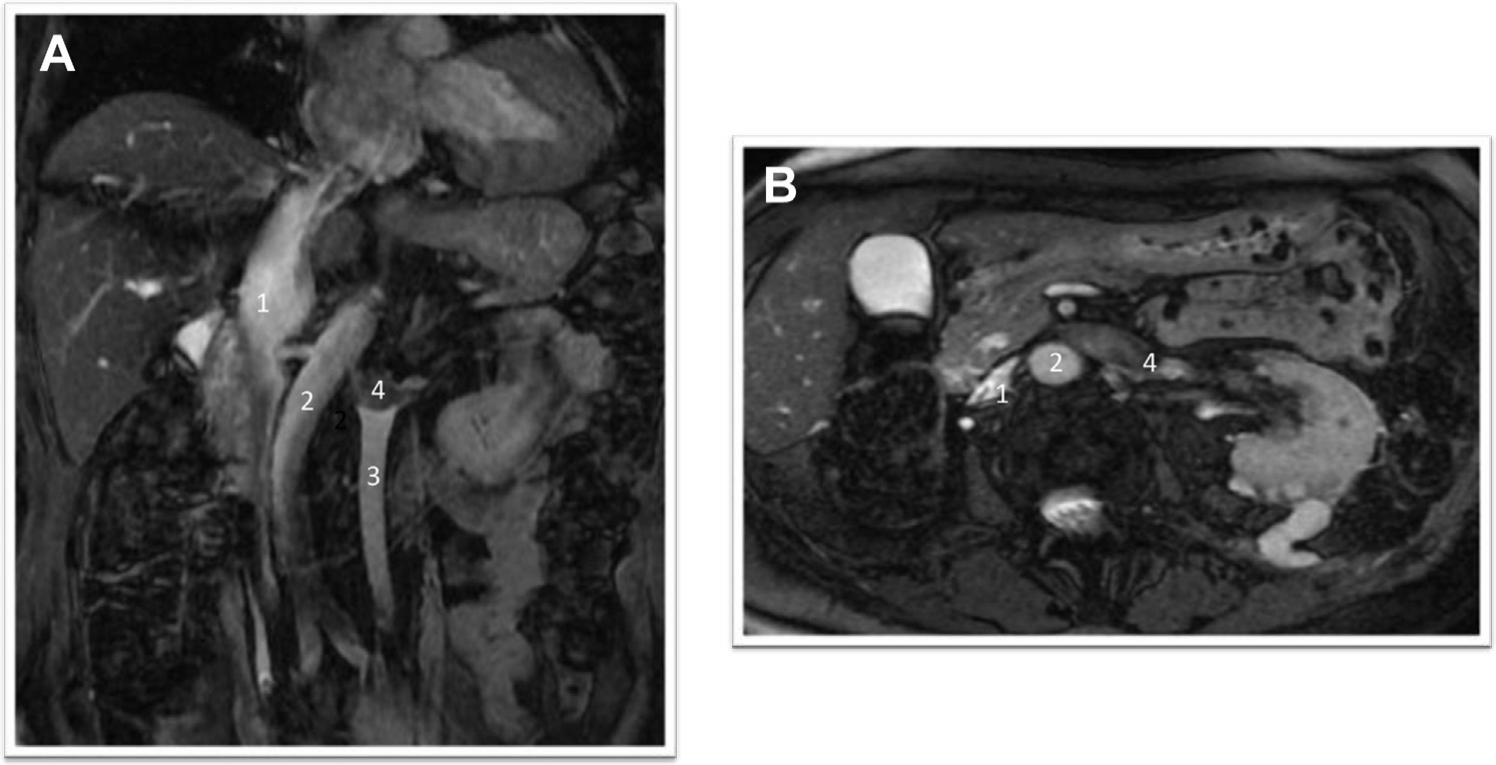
A, Coronal view of contrast-enhanced abdominal magnetic resonance image showing the right inferior vena cava (IVC; *1*), abdominal aorta (*2*), and left IVC (*3*) draining into a left renal vein partially obstructed by tumor (*4*). B, Axial view of contrast-enhanced abdominal magnetic resonance image showing right IVC (*1*) and left renal vein (*4*) with tumor over the abdominal aorta (*2*).
